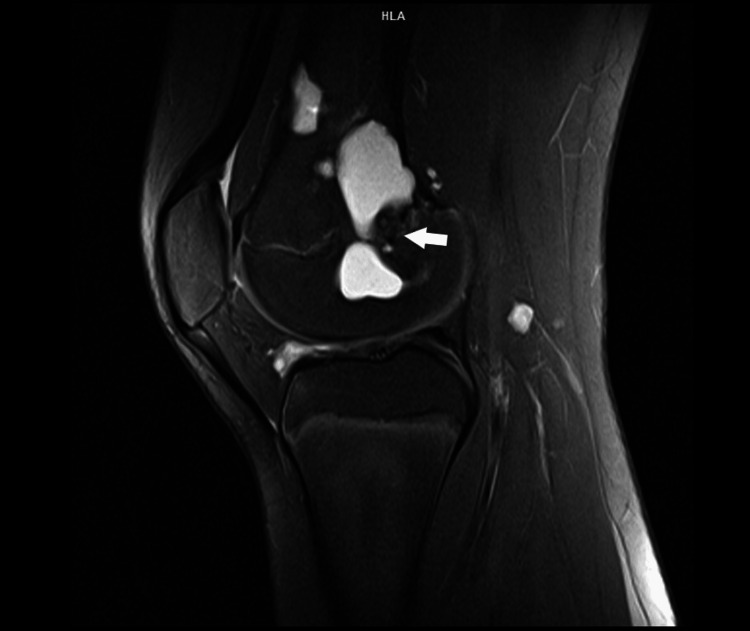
Sagittal T1 FS MRI image displays the reduced diameter of the remaining elements of the cyst, along with the disturbance of the normal line of the growth plate. FS: Fat saturation.
